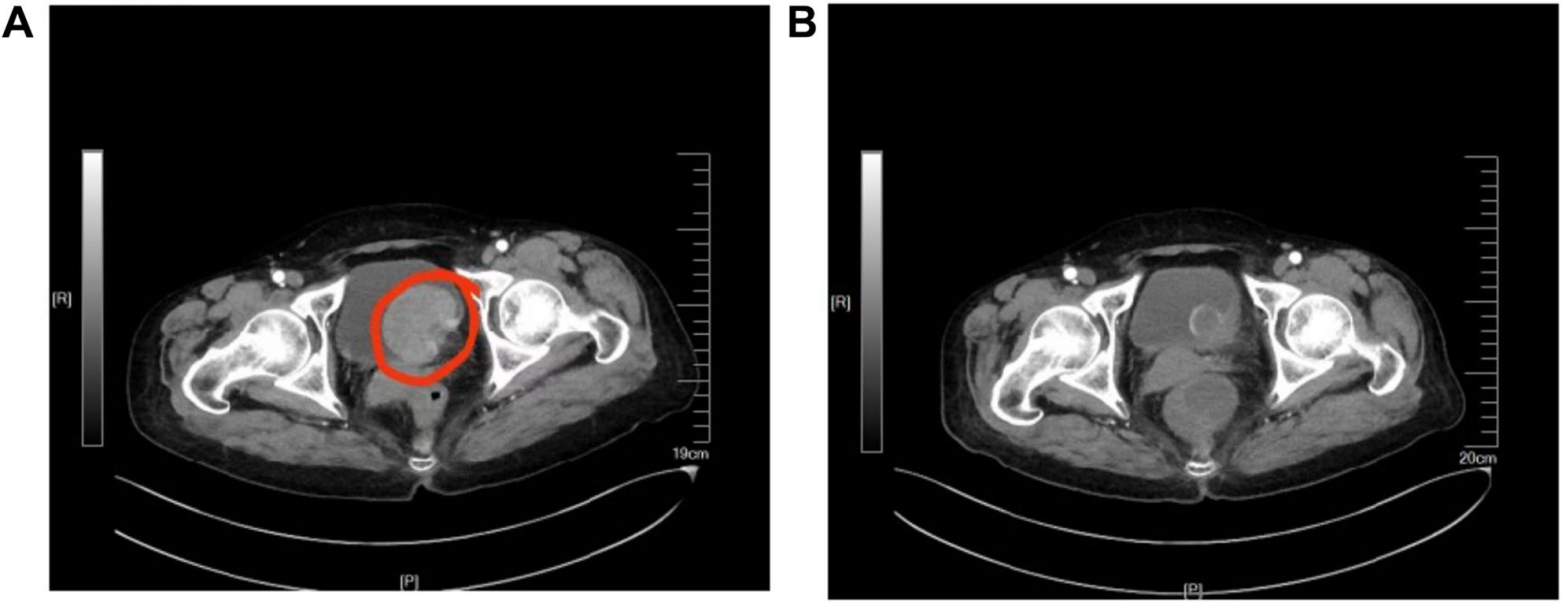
Contrast-enhanced CT imaging of the bladder before and after neoadjuvant therapy. A Baseline imaging: Axial CT image performed on November 29, 2024, prior to the initiation of neoadjuvant therapy, demonstrated a large, irregular mass (indicated by “O”) arising from the left posterior bladder wall. B Post-treatment imaging: Axial CT image performed on March 10, 2025, following completion of neoadjuvant therapy with tislelizumab and disitamab vedotin, showed significant regression of the previously noted mass, with only minimal residual soft tissue thickening or scarring at the primary tumour site
